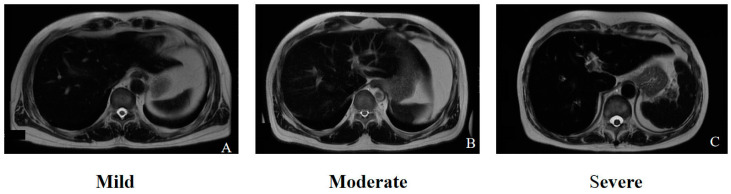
The T2-weighted sequences in (A–C) correspond to the respective severity levels (mild, moderate, and severe) of liver iron overload in the liver parenchyma.
